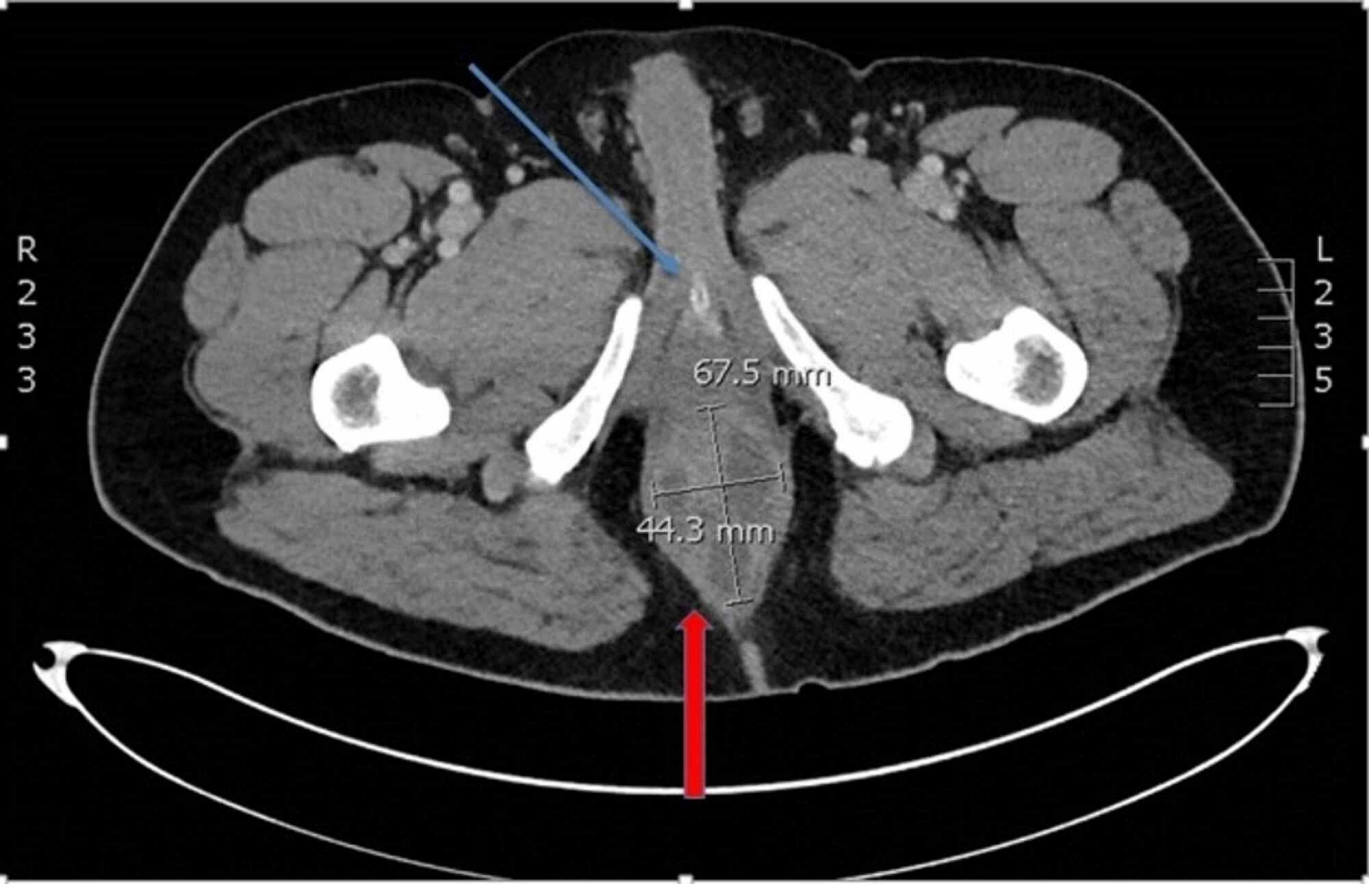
Transverse Slice of CT Pelvis with IV Contrast Ring enhancing lesion posterior to rectum (red arrow) and foley catheter (blue arrow).
